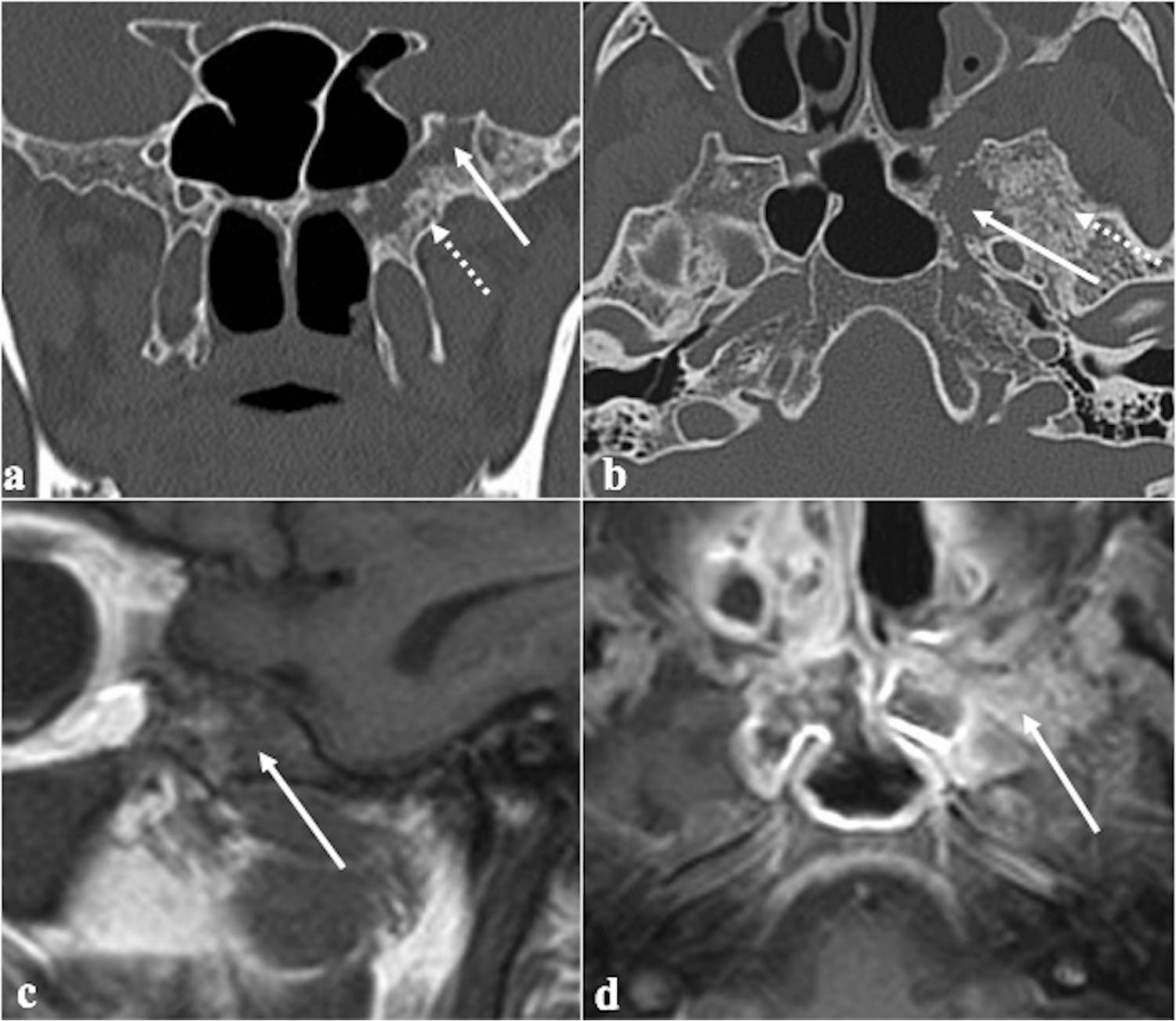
Sphenoid localization of multiple myeloma in an 82-year-old male patient. Coronal (a) and axial (b) CT images show a lytic lesion in the left greater sphenoid wing (white arrows) surrounded by bone sclerosis (white dotted arrows). MRI reveals a lesion in the left greater sphenoid wing characterised by low SI on sagittal T1W image (c, white arrow) and vivid enhancement after intravenous gadolinium contrast agent injection on axial T1W fat-saturated image (d, white arrow)
